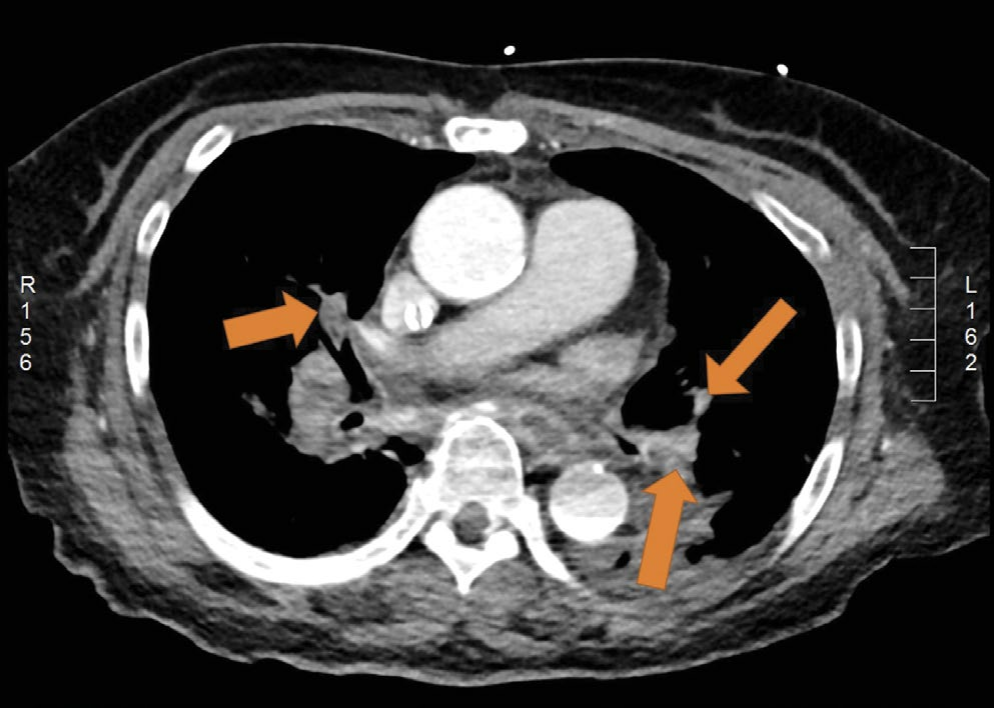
Chest computed tomography (CT) showing multiple pulmonary emboli (arrow)
